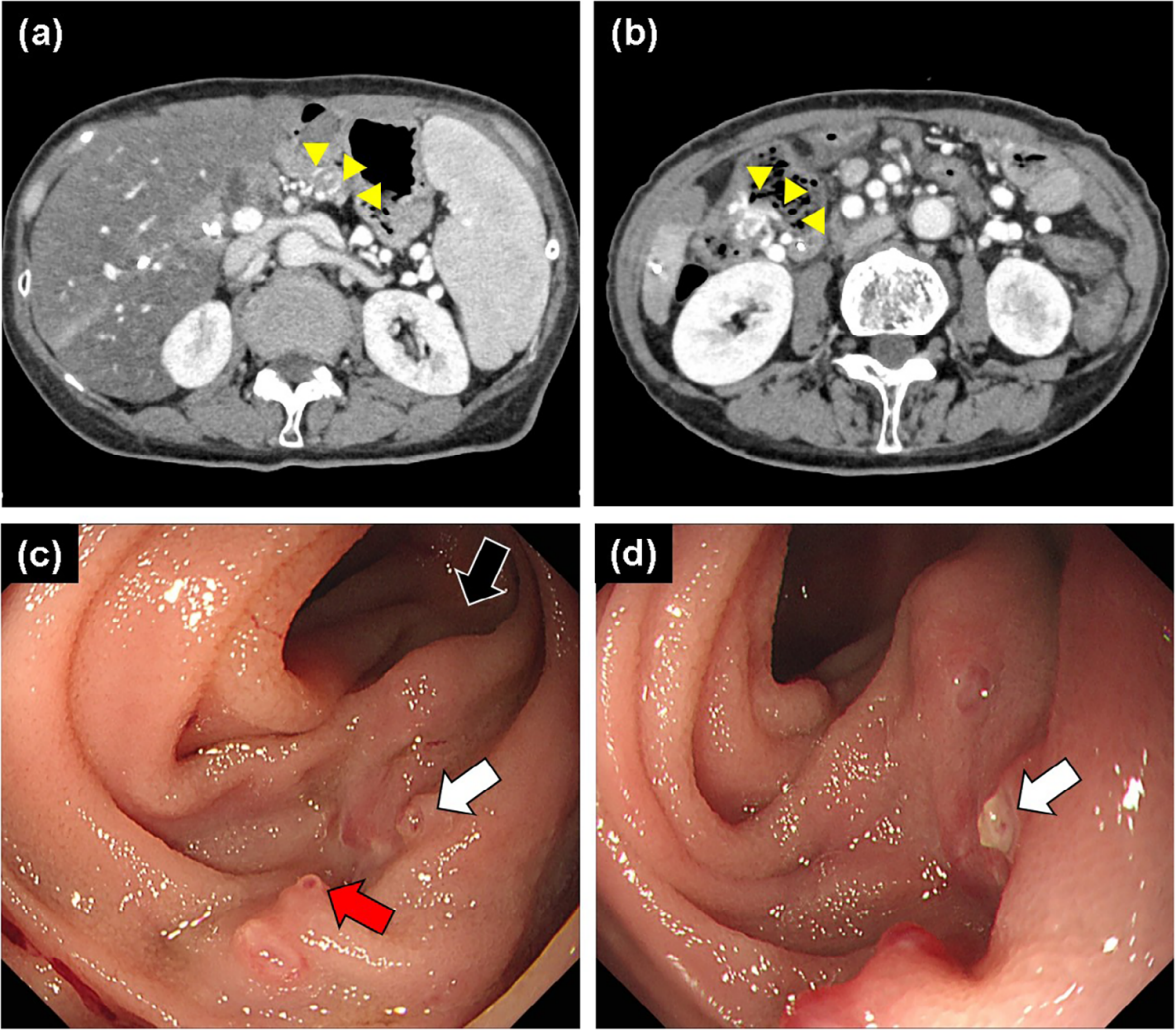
(a, b) Computed tomography: (a) pancreatojejunostomy varices (yellow arrowhead), (b) colonic varices (yellow arrowhead), (c, d) single‐balloon endoscopy reveals F2 varices with red color signs (red arrow) and white plugs (white arrow) around the pancreatojejunal anastomosis (black arrow).
